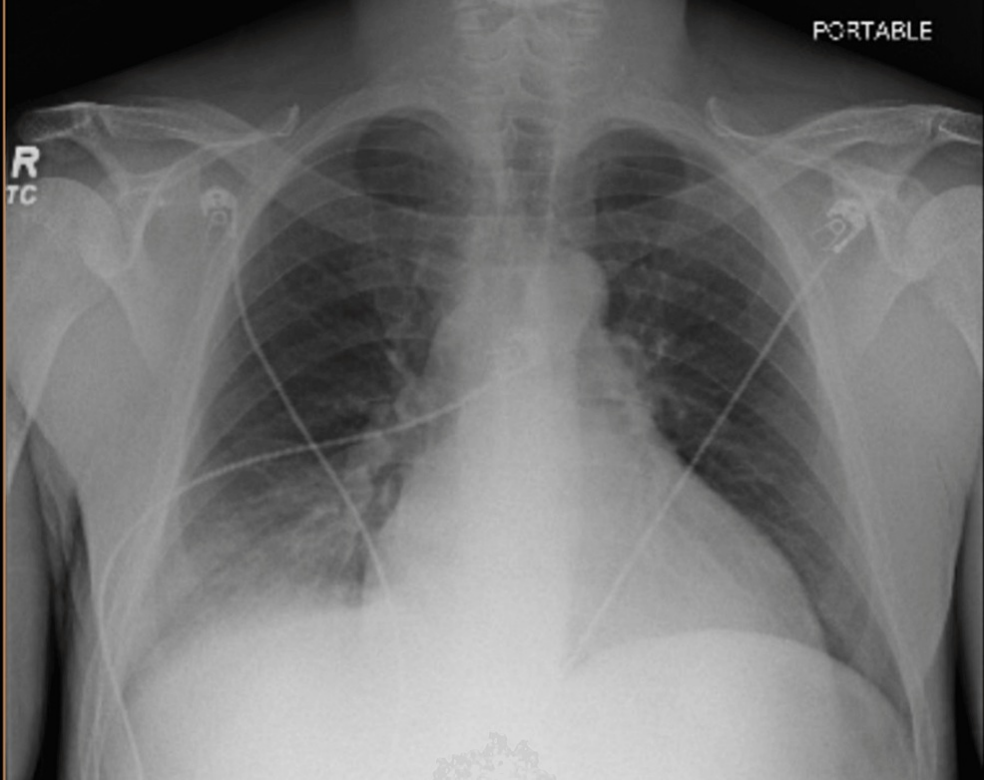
A portable chest radiograph demonstrating cardiomegaly and a right lower lobe opacity
